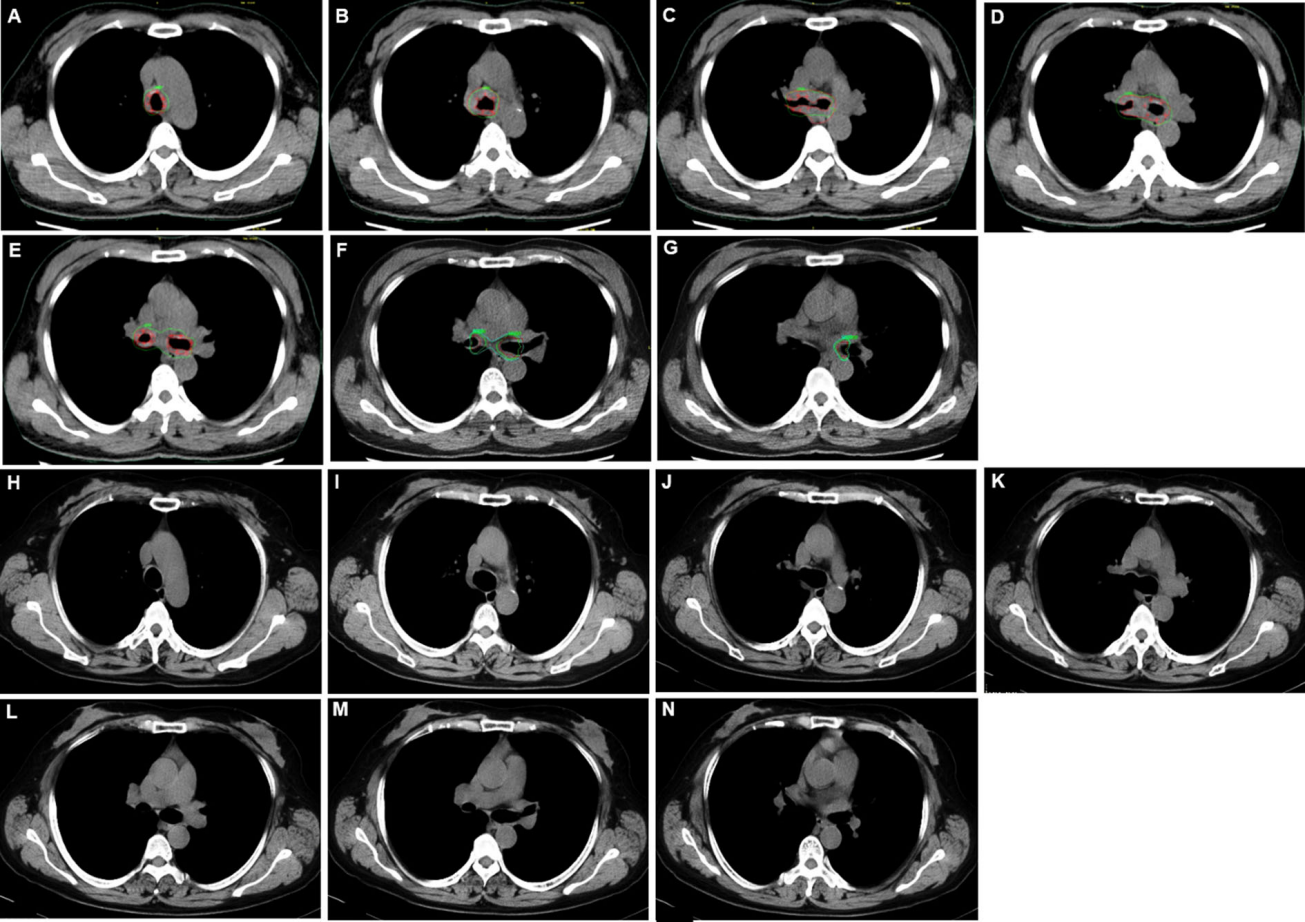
Thoracic computerized tomography (CT) scans for adenoid cystic carcinoma (ACC) in central airway. (A–G) Thoracic CT scans before placing the stent with ^125^I seeds. (H–N) Thoracic CT scans 35 months after removing the stent with ^125^I seeds.
